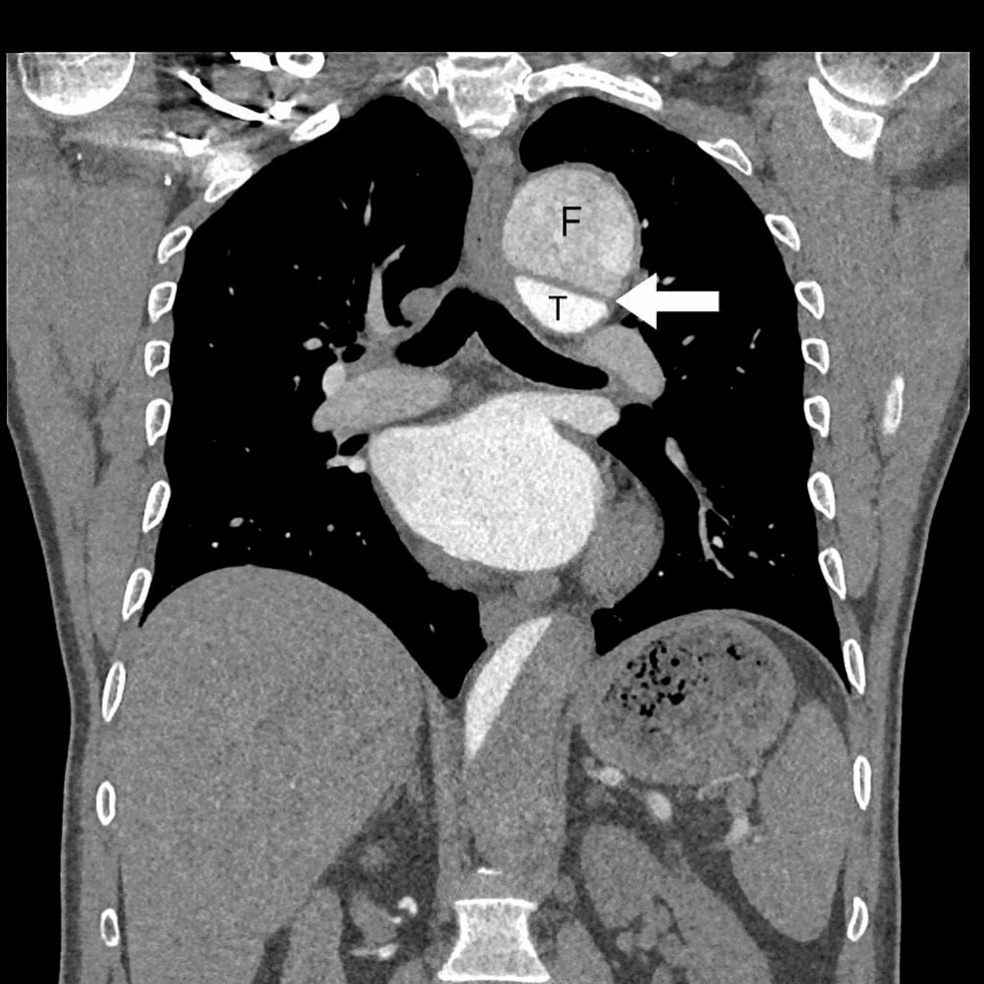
CT angiogram of the chest, coronal view, demonstrating an intimal flap (white arrow) in the descending aorta with the formation of true and false lumens
